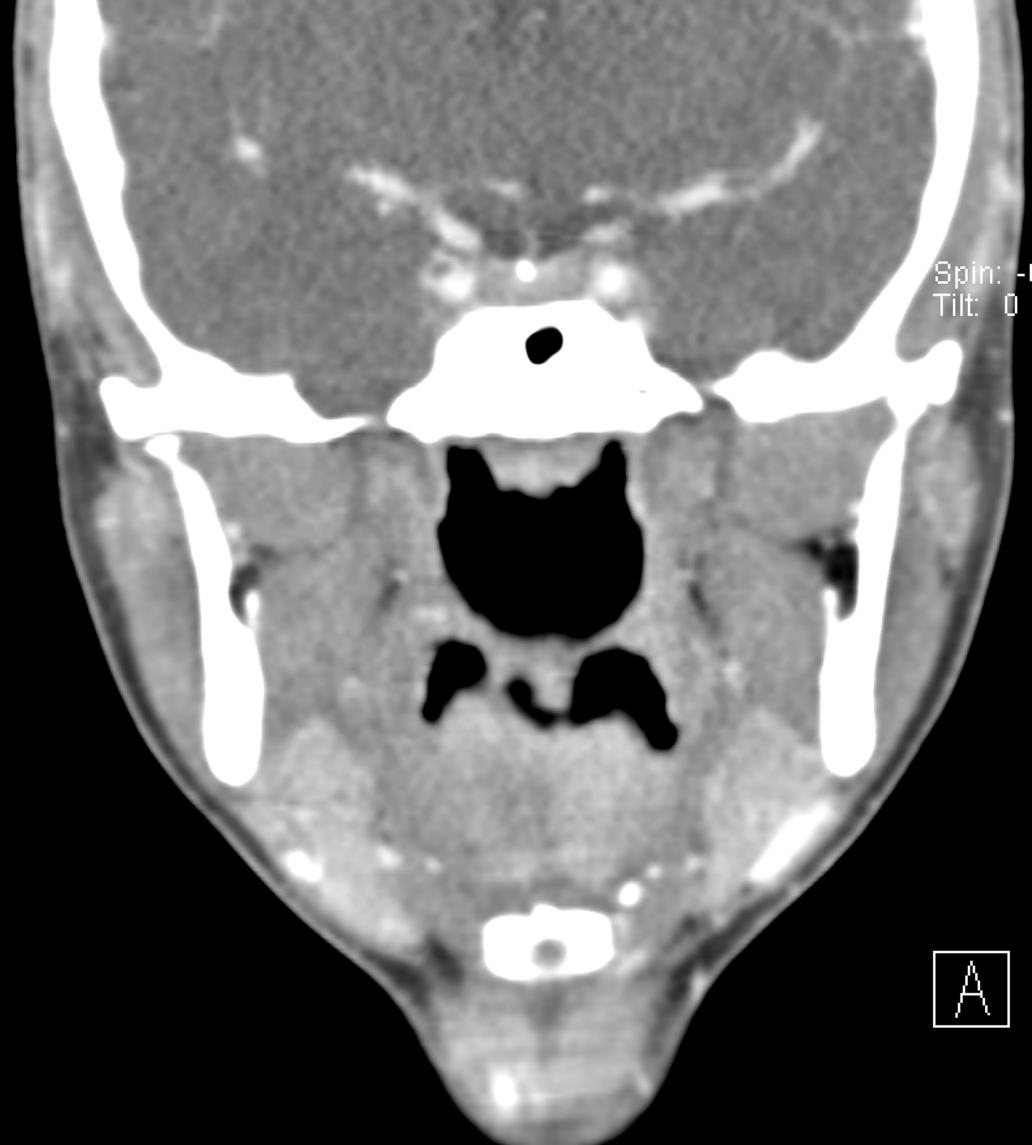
Coronal non-contrast maxillofacial computed tomography (CT) (soft tissue window) demonstrates no soft tissue abnormality or mass
